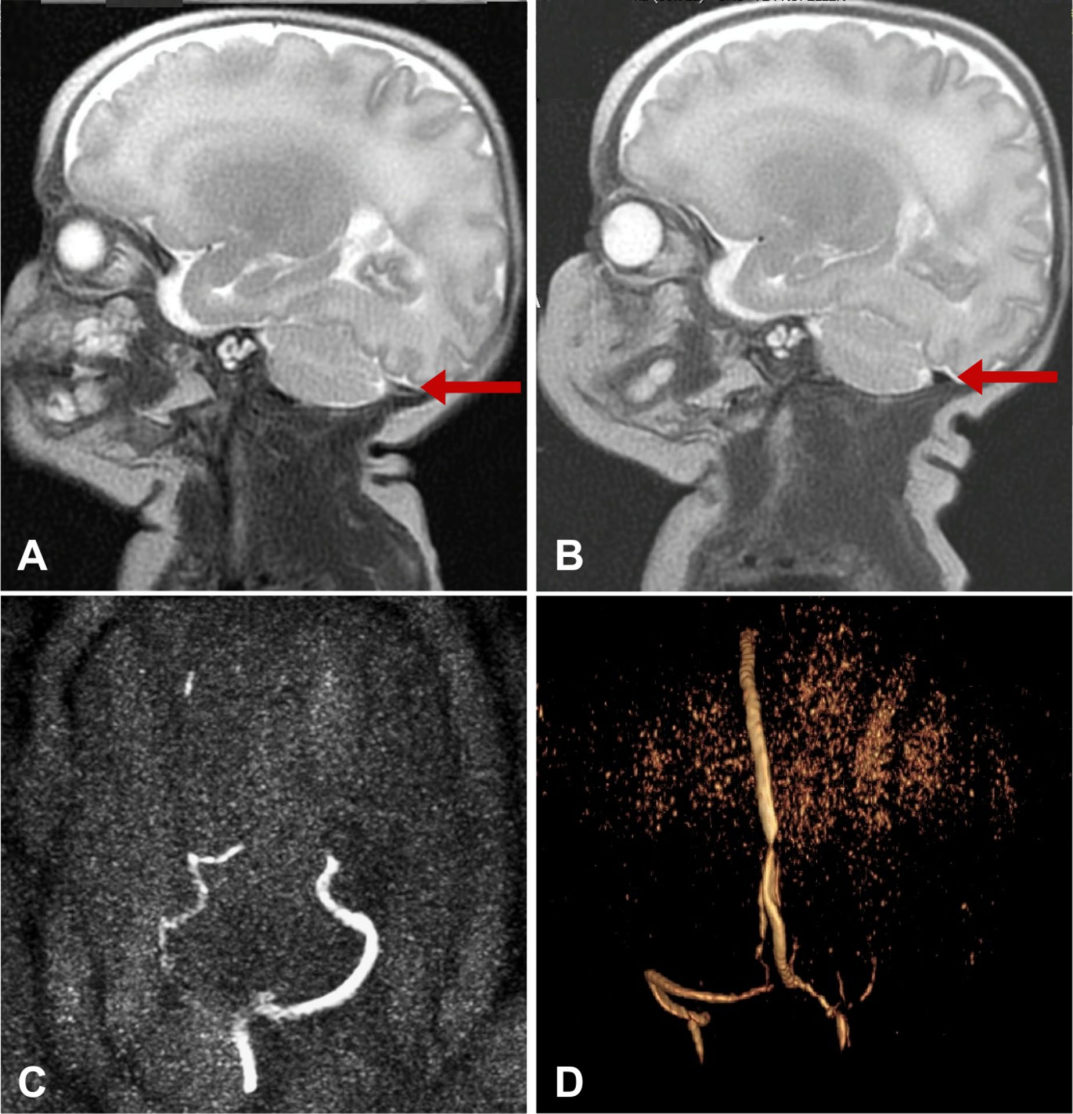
Magnetic resonance imaging. A MRI scan, performed on the 82th day of life, right sagittal cross-section, red arrow points to transverse sinus. B MRI scan, performed on the 82th day of life, left sagittal cross-section, red arrow points to transverse sinus. C, D MR venography with visible flow in both transverse sinuses, markedly smaller on the right side
